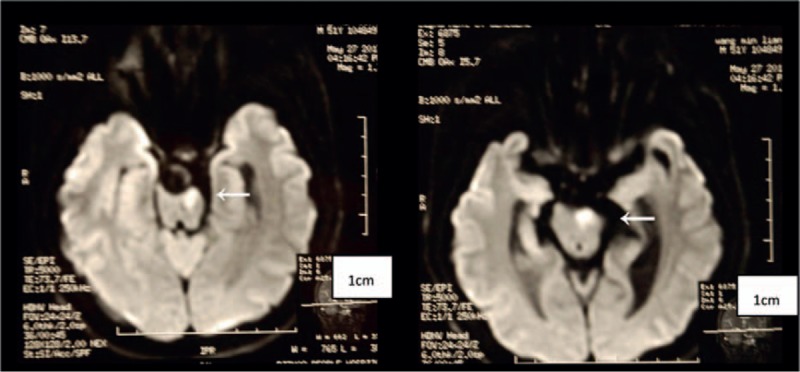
High-intensity signals on DWI indicating an acute pontine infarction.
